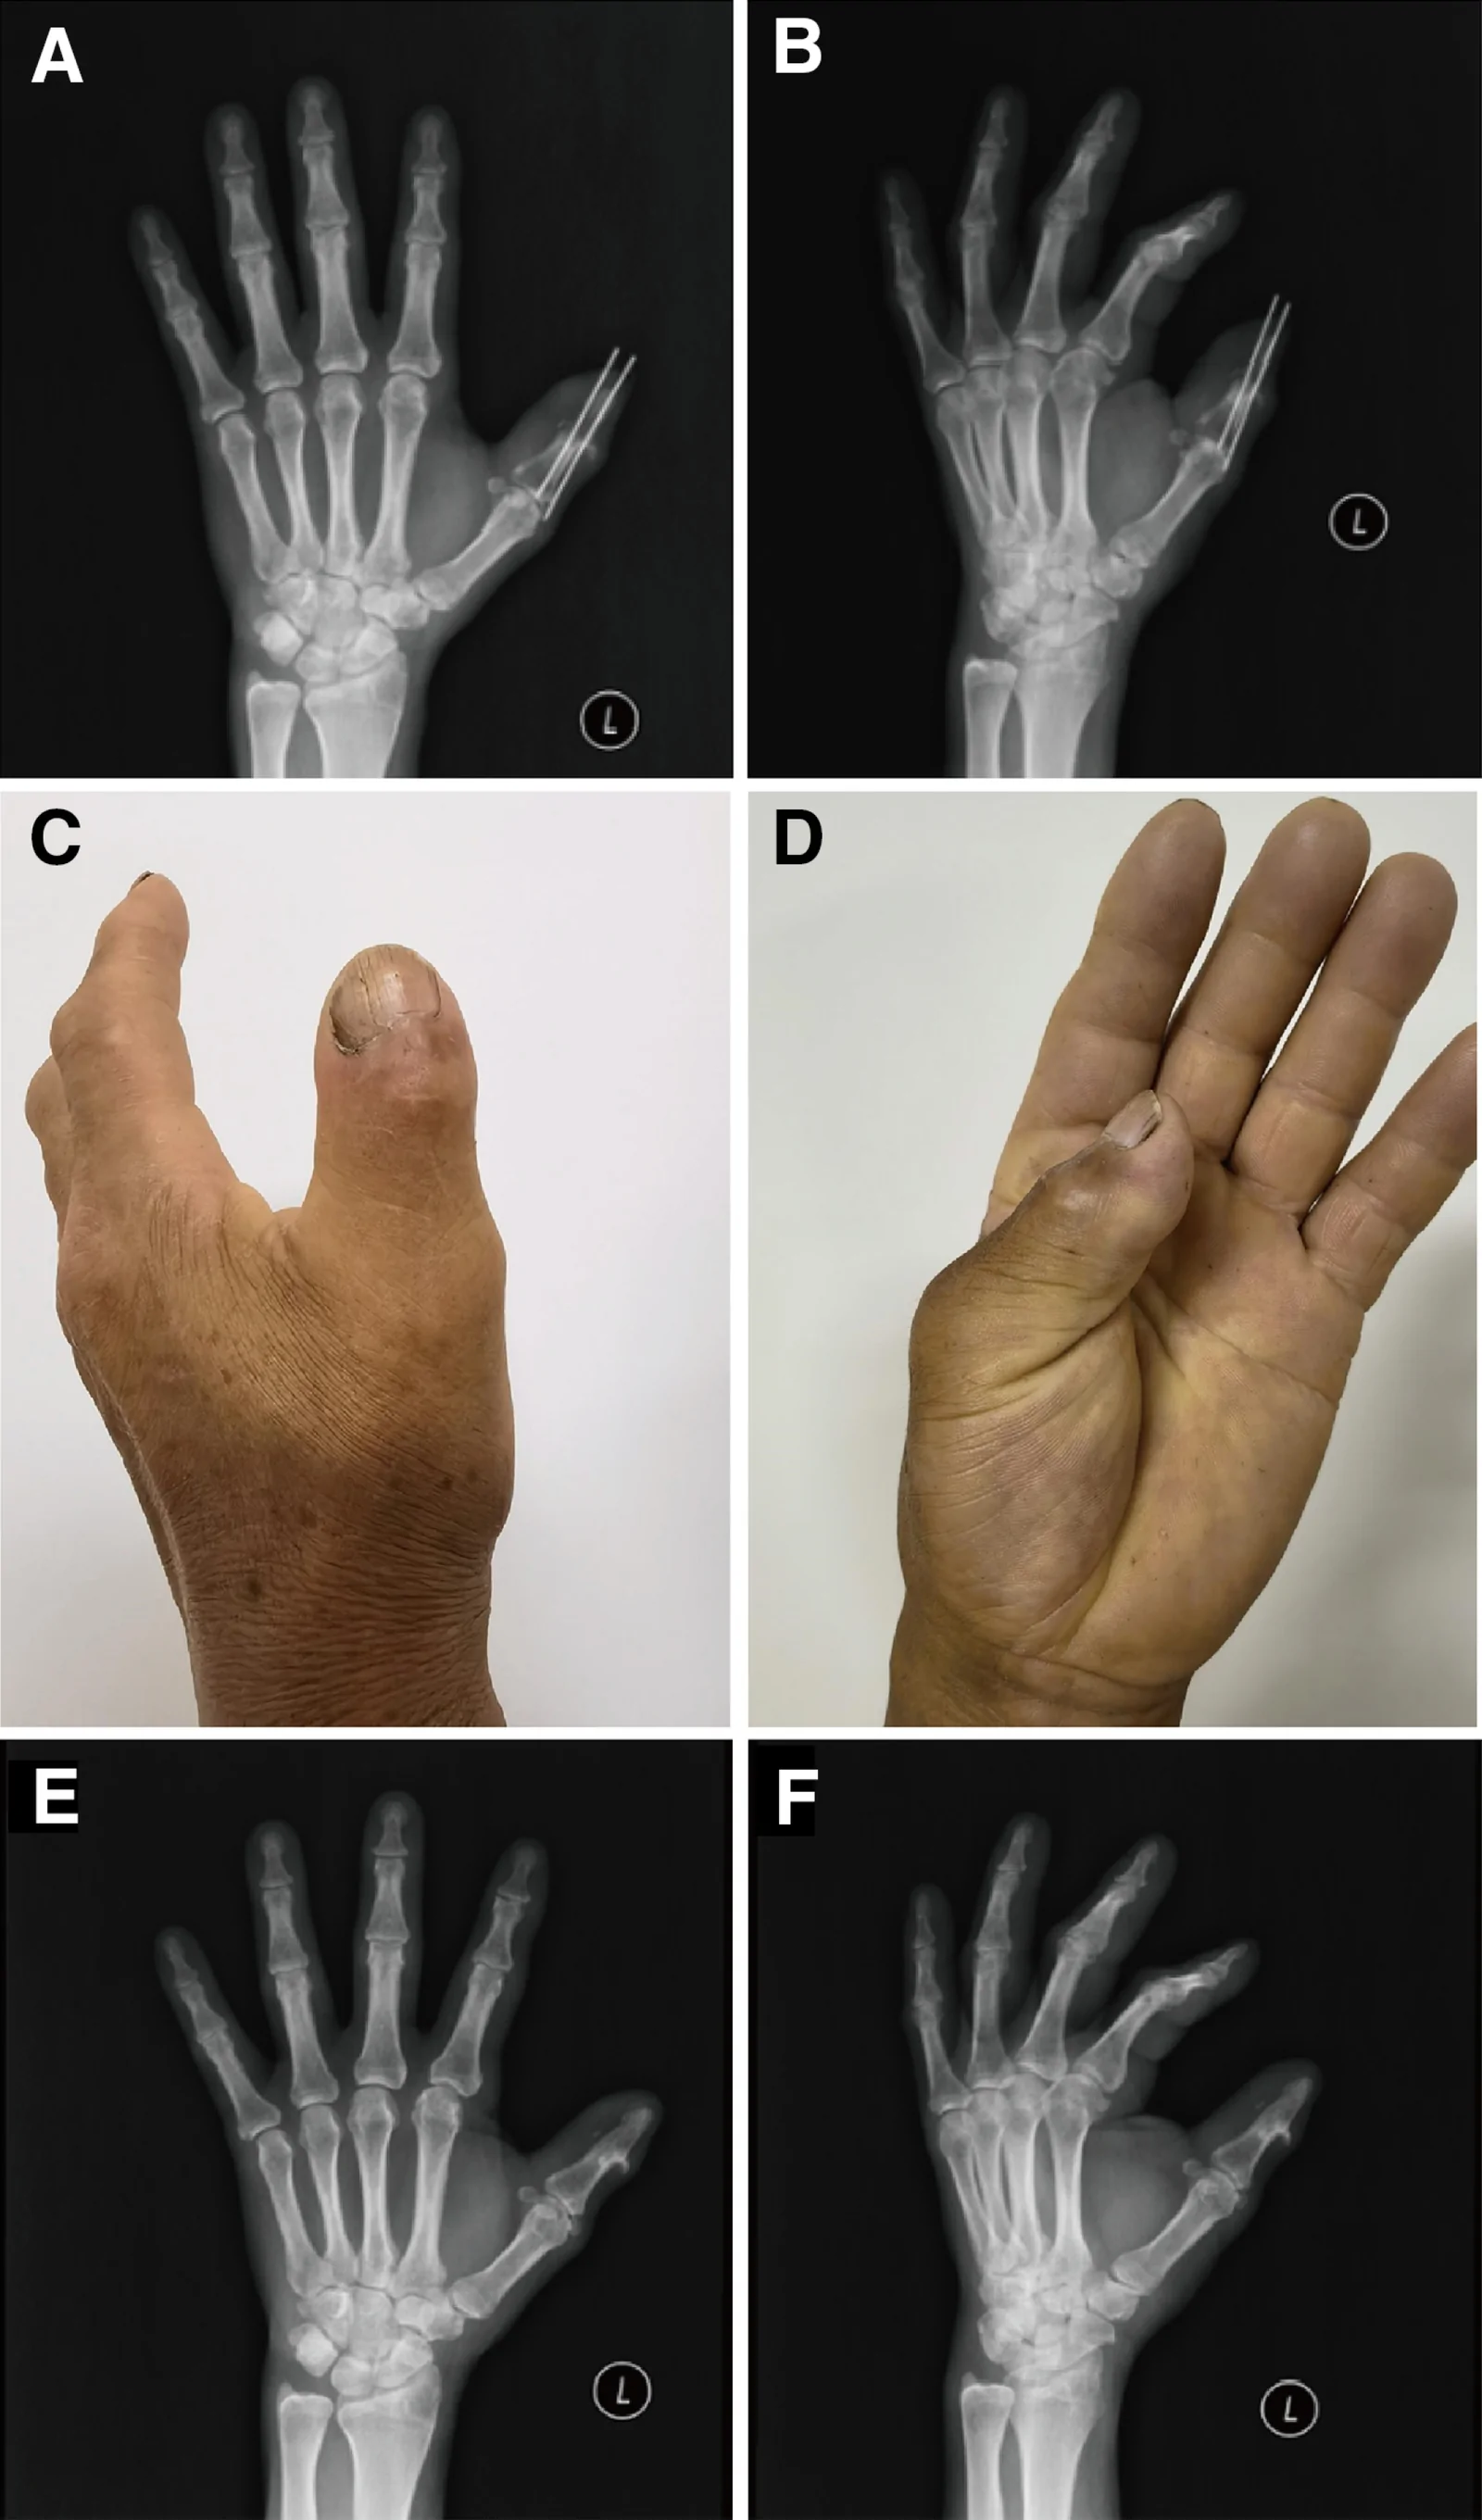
One-month postoperative anteroposterior (A) and lateral (B) X-rays of the left hand. Six-month postoperative photos (C,D) of the patient’s left hand. Six-month postoperative anteroposterior (E) and lateral (F) X-rays of the left hand.
